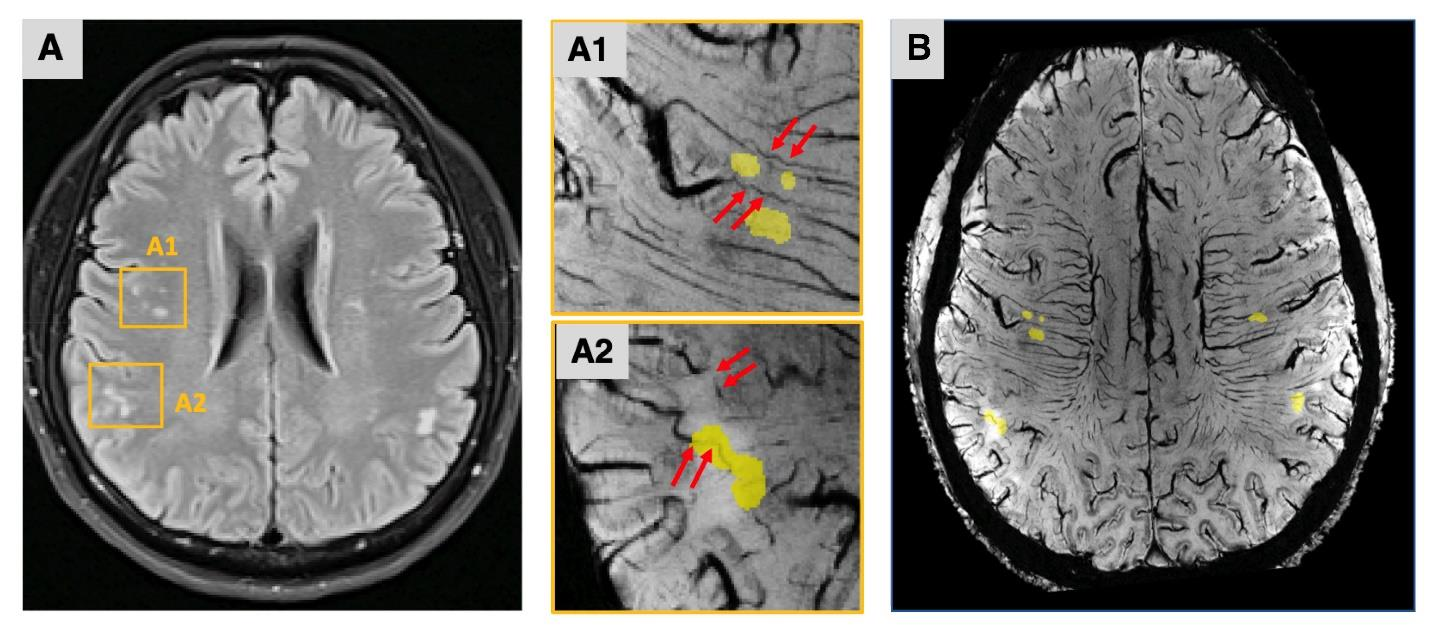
Spatial relationship between tortuous arteries and white matter lesions. (A) White matter lesions were revealed on FLAIR. A1 and A2 were magnified panels of lesions overlaid on the post-contrast 3D susceptibility-weighted imaging (SWI) data. The WM lesions (yellow masks) were adjacent to or at the distal end of the tortuous arteries (red arrows). (B) After linear registration, the lesion mask was overlaid on the post-contrast SWI (mIP = 2) images. The ischemic leukoaraiosis located in areas where the cerebral small arteries (i.e., medullary arteries) provide the blood supply.
